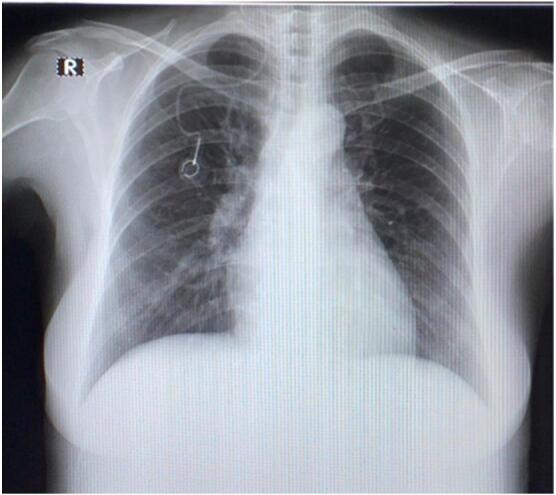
Thoracic X-ray post-TIVAP insertion.
